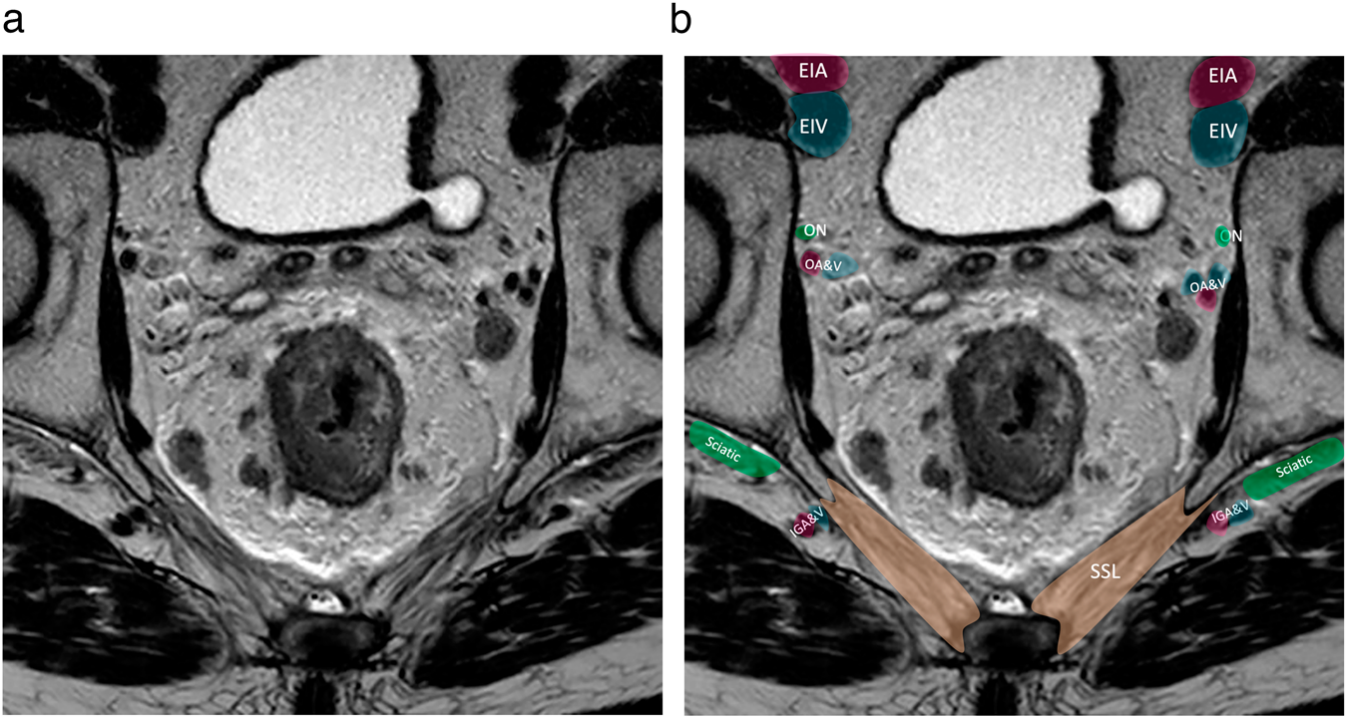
Axial T2-weighted MR image (a without annotations, b with annotations) at the level of the S5, pointing out the position of the obturator nerve which is a very good surgical landmark, especially for lymphadenectomy. SSL, sacrospinous ligament; IGA&V, inferior gluteal artery and vein; OA&V, obturator artery and vein, ON, obturator nerve; EIA, external iliac artery; EIV, external iliac vein
